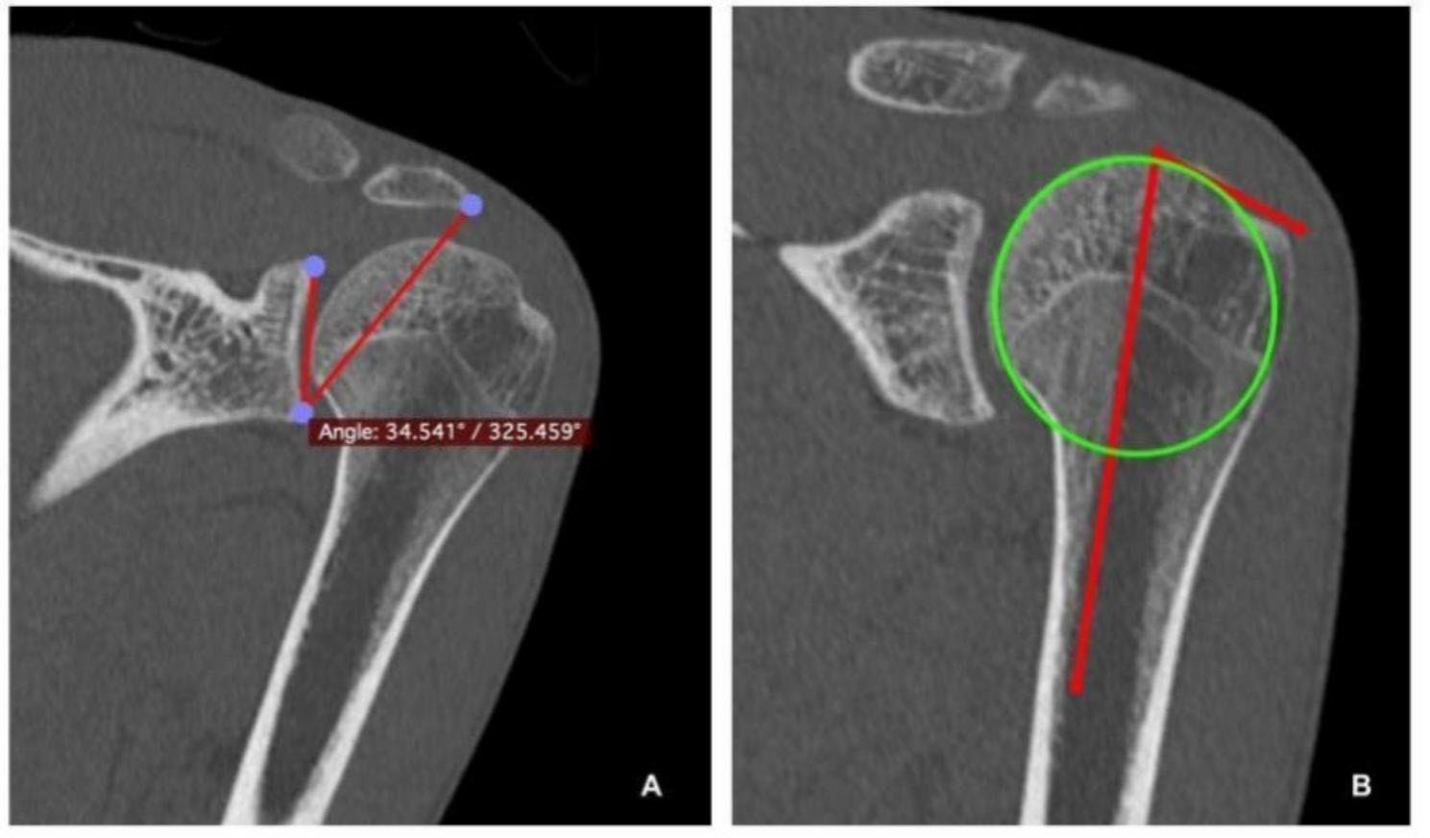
3D corrected CT-scan reconstruction of a left shoulder showing the assessment of CSA and GTA. (A) CSA is based on the angle between the glenoid plane and most lateral border of the acromion. (B) GTA consists of the angle between a parallel line to the diaphyseal axis that passes through the humeral head center of rotation and the most superolateral edge of the greater tuberosity.
